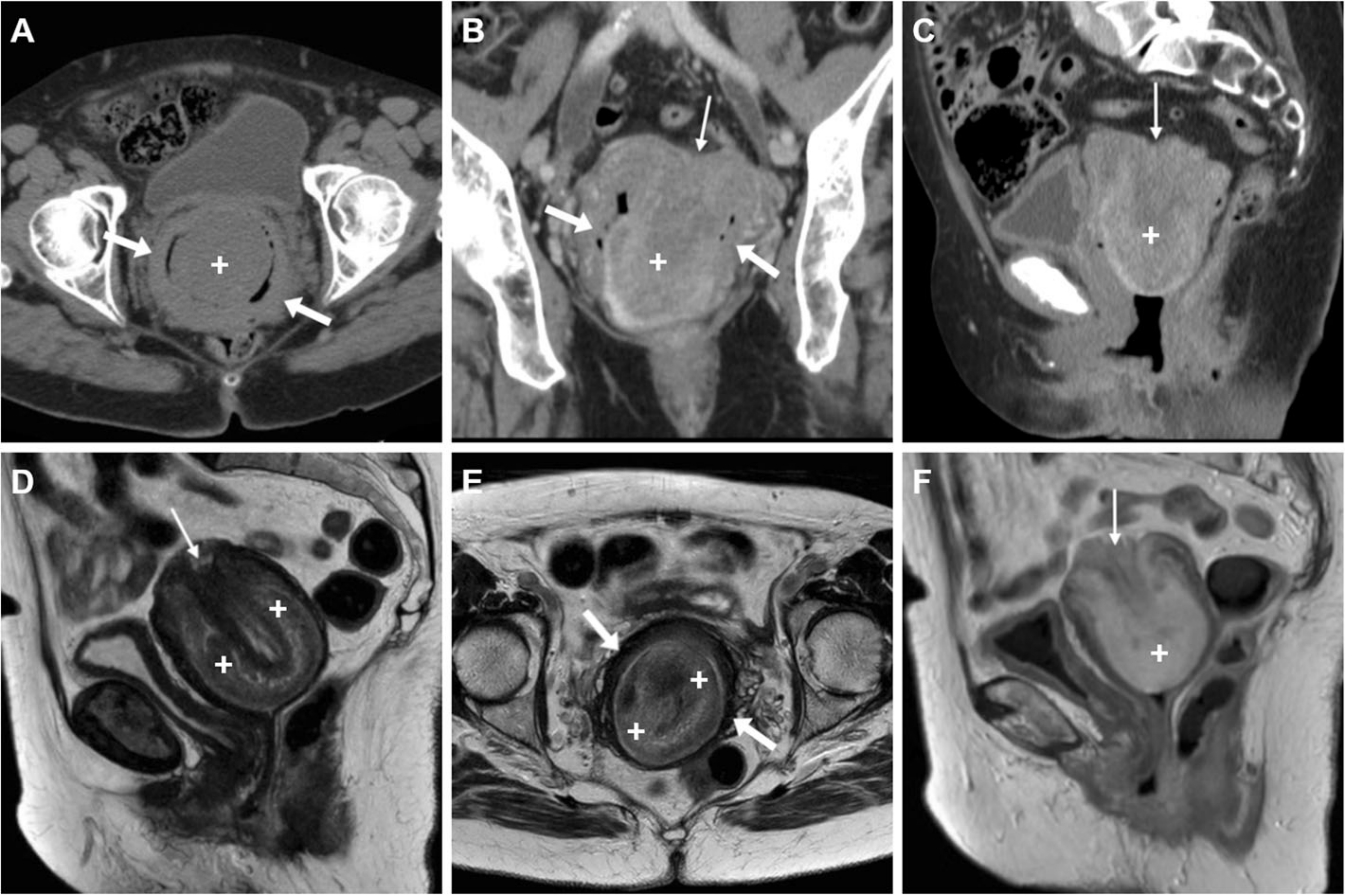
Surgically confirmed, spontaneous uterine inversion without mass lesions in a 79-year-old woman experiencing muco-haemorrhagic vaginal discharge. Precontrast (a) and contrast-enhanced (b, c) CT images showed a solid, mass-like enhancing structure (plus sign) surrounded by air coursing through the dilated uterine cervix. Loss of the normally convex uterine fundus (thin arrows) was noted. Physically, the upper vagina was occupied by the inverted uterus. Sagittal (d) and axial (e) T2-weighted MRI images confirmed the diagnosis by showing lost convexity and depression of the uterine fundus (thin arrows), U-shaped inverted uterus (plus sign) with preserved zonal anatomy for age and normal homogeneous enhancement on post-gadolinium T1-weighted image (f), which coursed downwards through the hypointense uterine cervix walls (thin arrows) [adapted from Open Access ref. no [33]]
